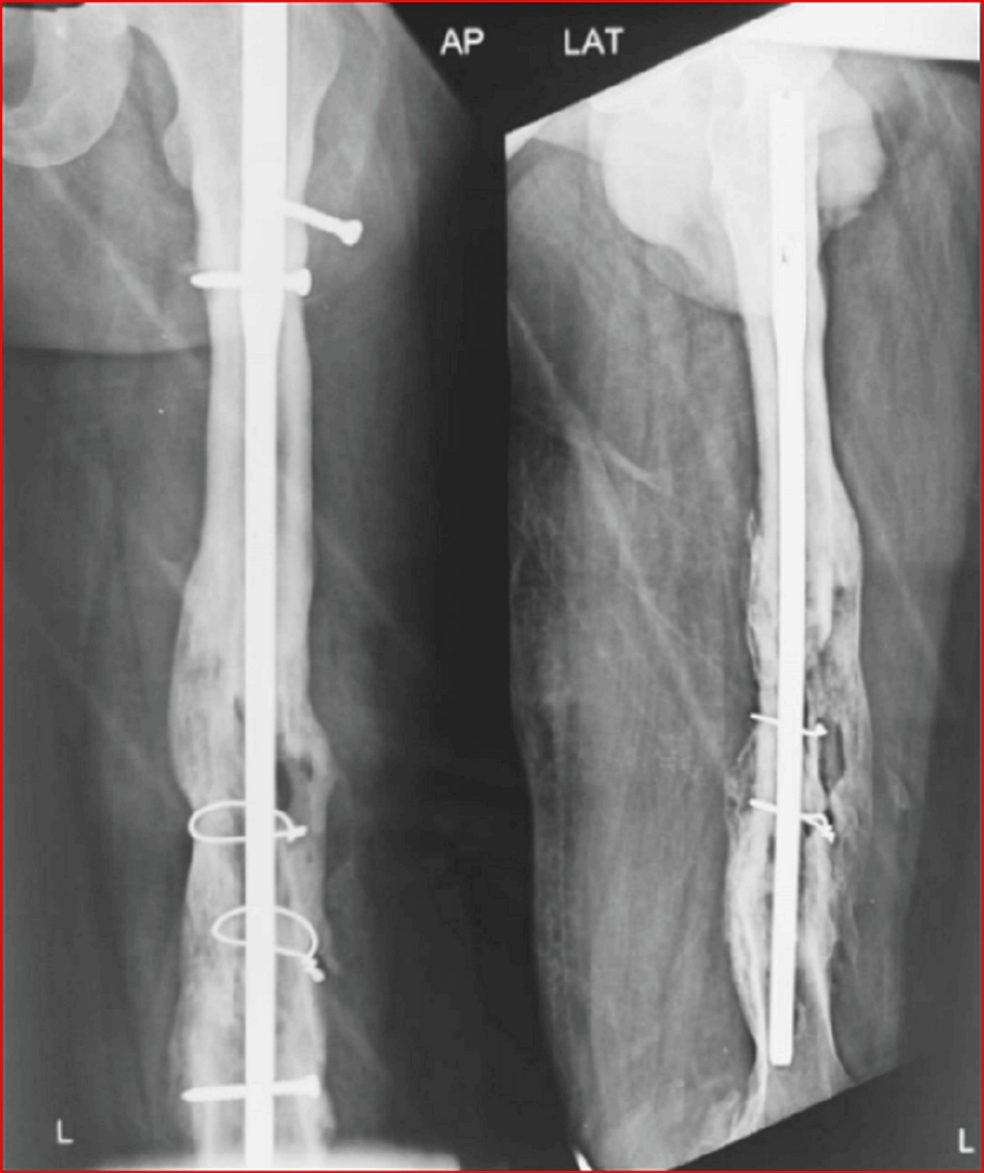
X-ray of the united femur fracture with an interlock nail, loose screws, cerclage wires and evidence of infection of the bone
